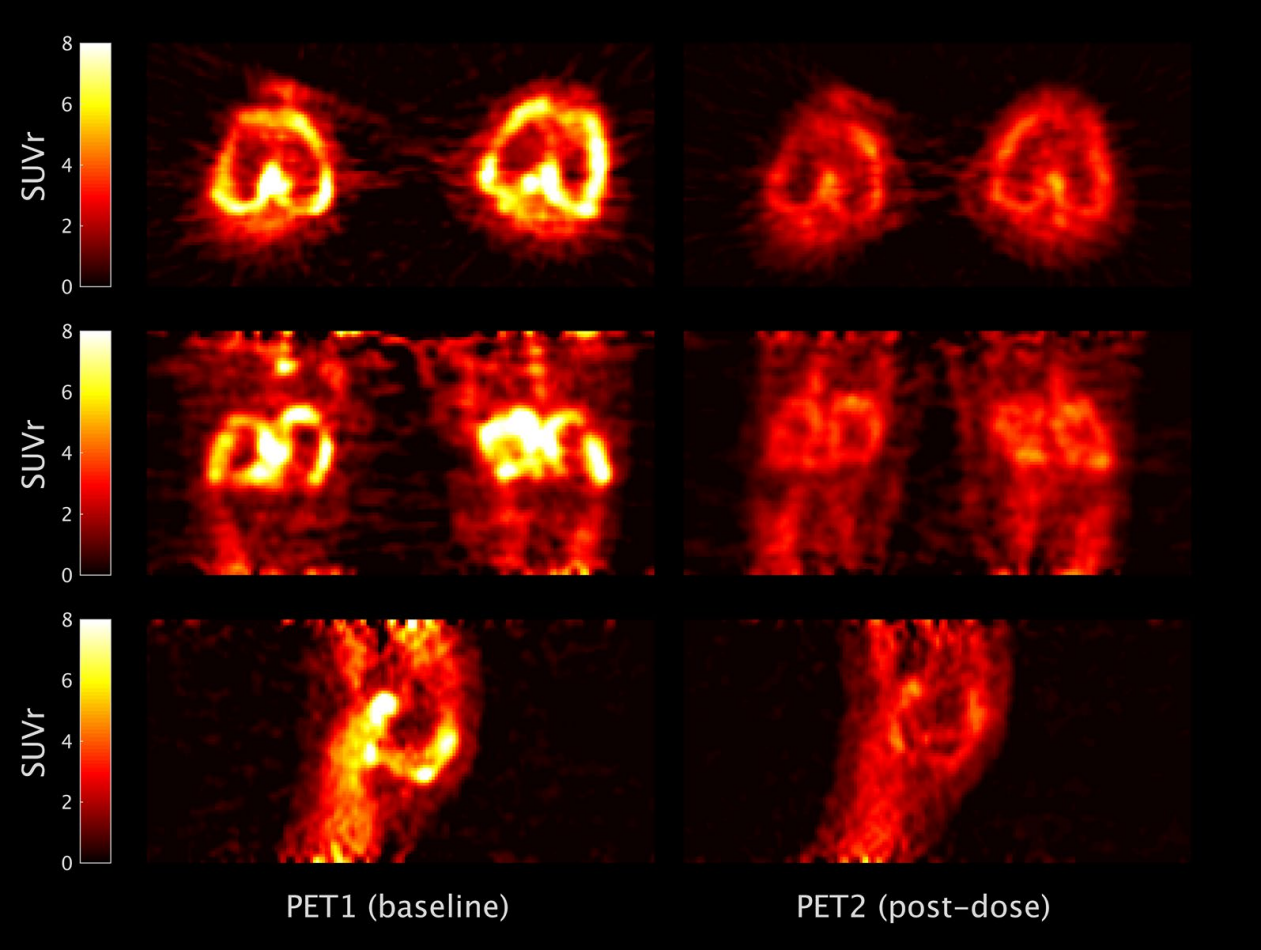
Trans-axial (top), coronal (middle) and sagittal (bottom) images of [^11^C]PBR28 uptake normalized for blood activity (SUVr_50–70_ blood) in subject 2 shows synovial uptake at baseline (PET1 baseline) that is reduced after administration of oral emapunil (PET2 post-block)
